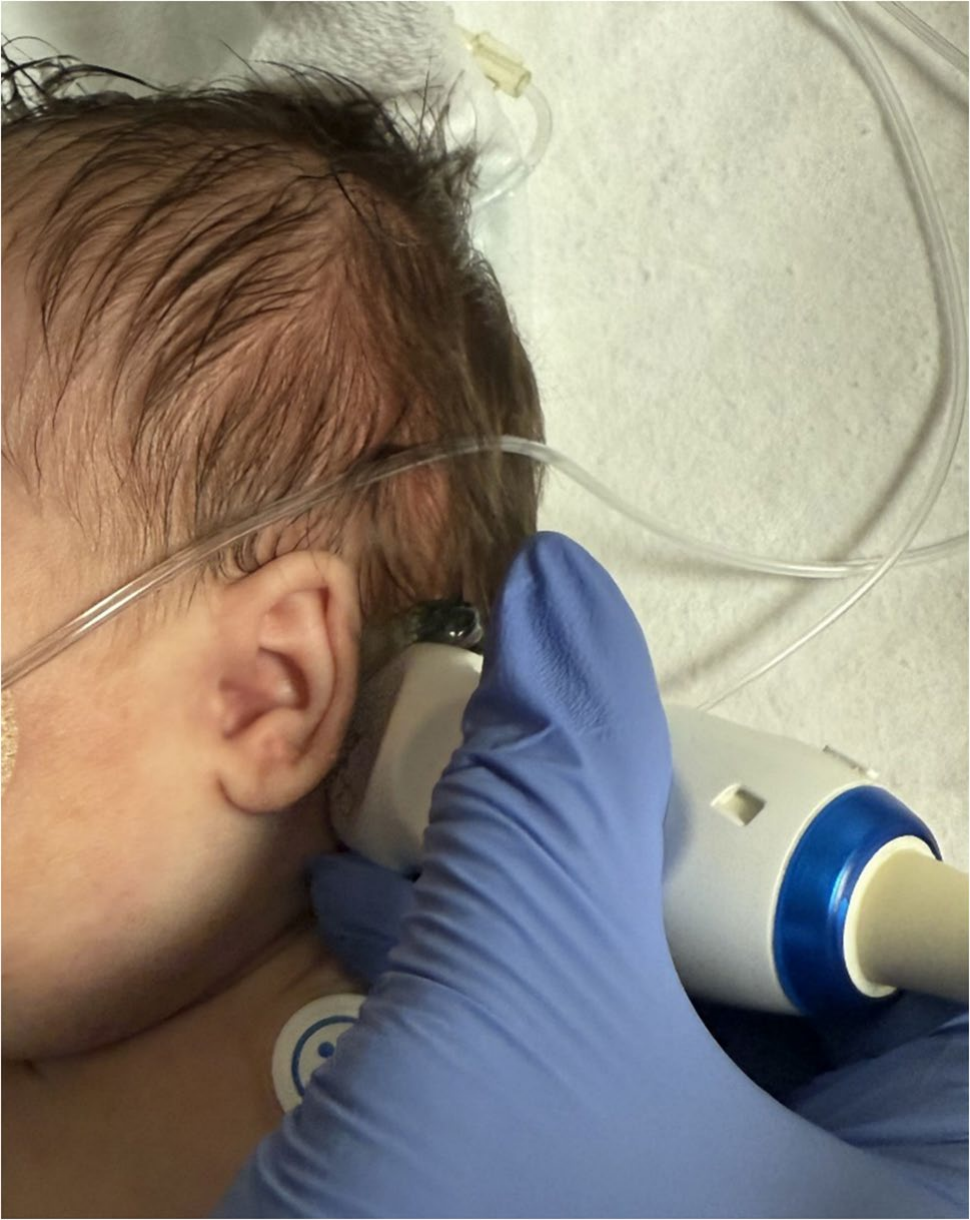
Transmastoid ultrasound being performed on a 10-day-old girl born at 34 weeks of gestation by placing a micro-convex array transducer on the mastoid fontanelle just behind the left ear. The transducer is swept and angulated to obtain a symmetrical image of the cerebellar hemispheres
